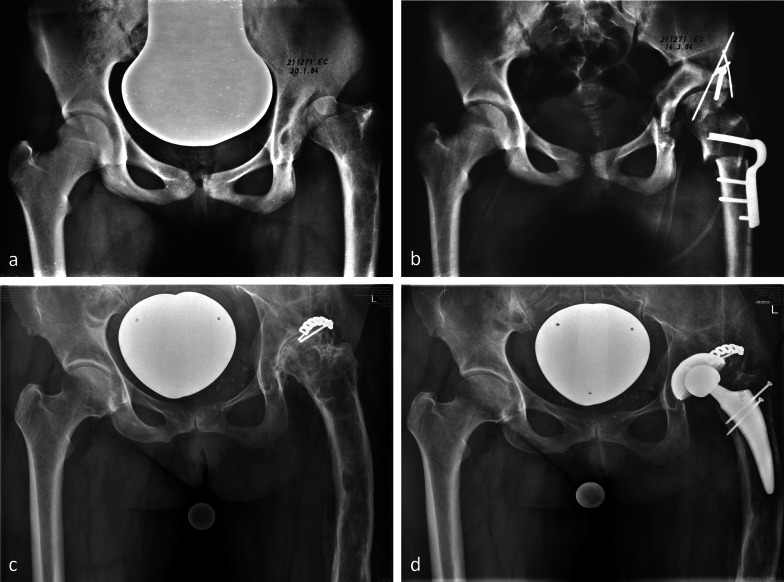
1984: first operated case—13-year-old female with PFFD of the left hip. a Varus proximal femur after intertrochanteric osteotomy 3 years earlier. b Periacetabular osteotomy followed by intertrochanteric revalgisation for better joint congruity. Good lateral coverage of the head. Increased retroversion of the acetabulum led to posterior subluxation 3 months after surgery, which was treated with a posterior shelf. c Progressive joint degeneration after 36 years. Plate for shelf fixation still in place. d Follow-up radiography of THR 2 years later. The patient regained a normal gait pattern
